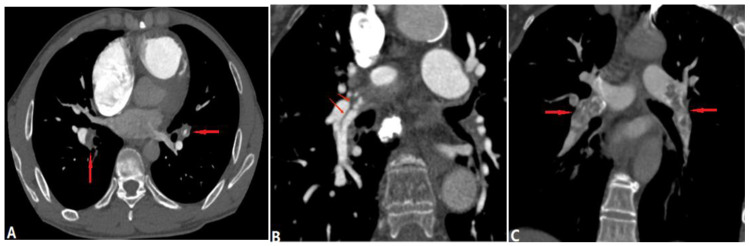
Chronic pulmonary embolism. (A): Axial CT image shows bilateral eccentric laminated filling defects and pulmonary artery stenosis (arrows) in a patient with chronic pulmonary embolism. (B): Coronal reconstruction CTA image in another patient with dyspnea shows a web (arrows) or band within a lower-right lobar pulmonary artery, consistent with chronic PE. (C): Coronal reconstruction CTA image in a patient with a history of acute PE shows calcified obstructive thrombus (arrows) within bilateral pulmonary arteries. CTA—computed tomography angiography; PE—pulmonary embolism.
